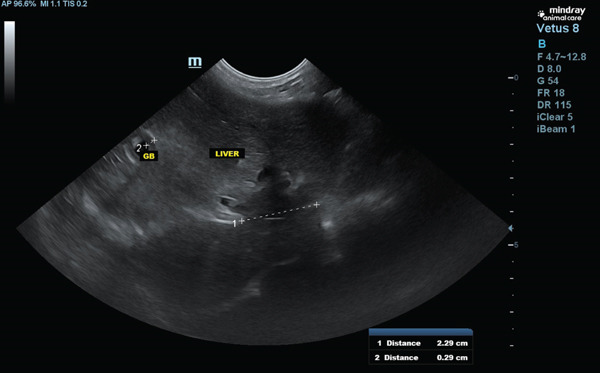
Ultrasound showing subxiphoid transverse hepatic approach, with slight cranial angulation.
